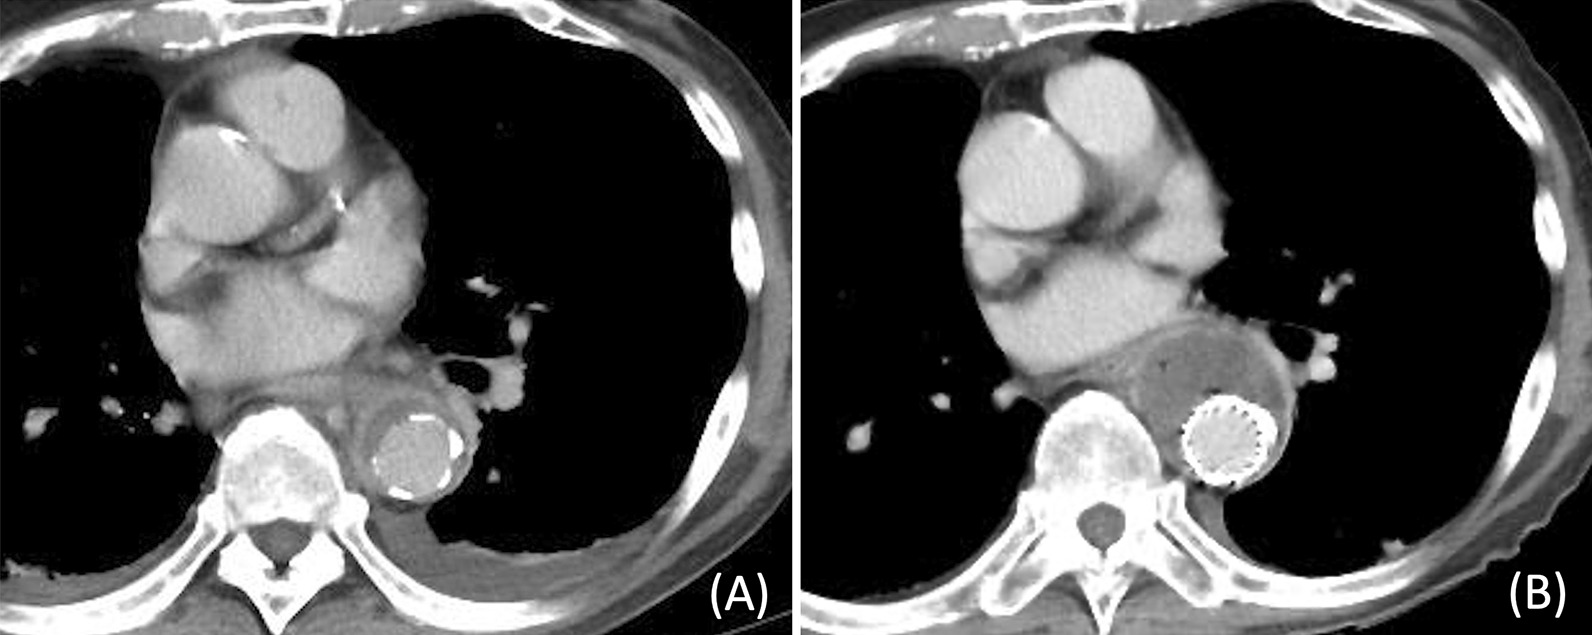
Despite antibiotic treatment after TEVAR, contrast-enhanced CT suggests abscess expansion over time (A: three days after the initial TEVAR; B: 29 days after the initial TEVAR)
